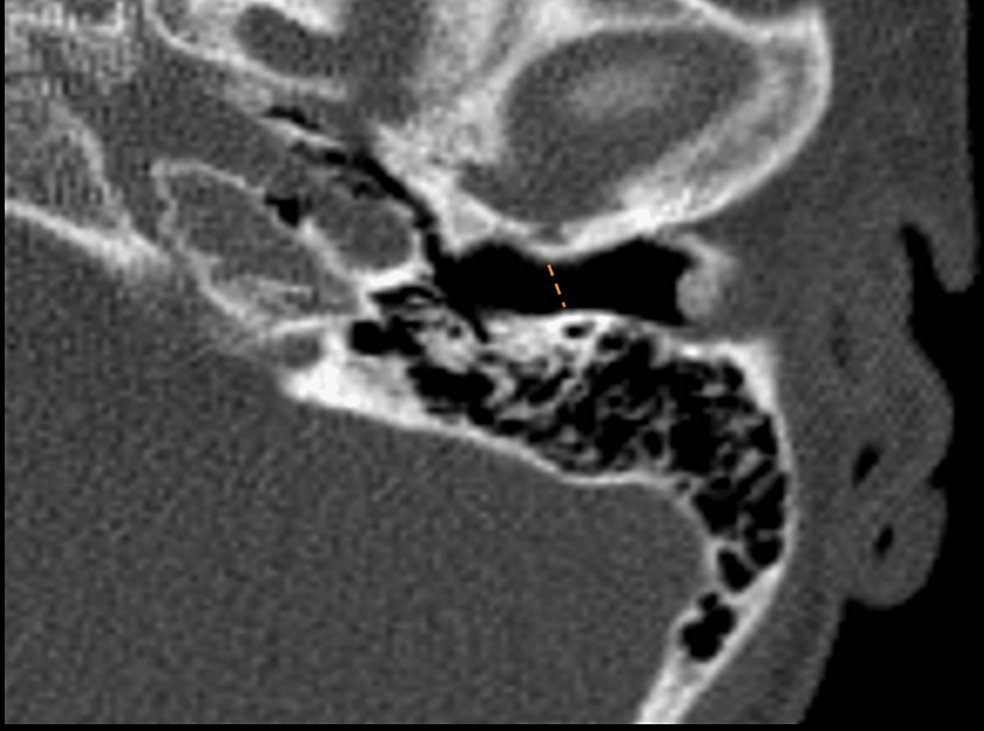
Axial cut of CT scans through the left temporal bone when the patient was age ten. Bony hypertrophy of the left external auditory canal (EAC) with lateral soft tissue obstruction and further canal stenosis is noted (dotted line).
